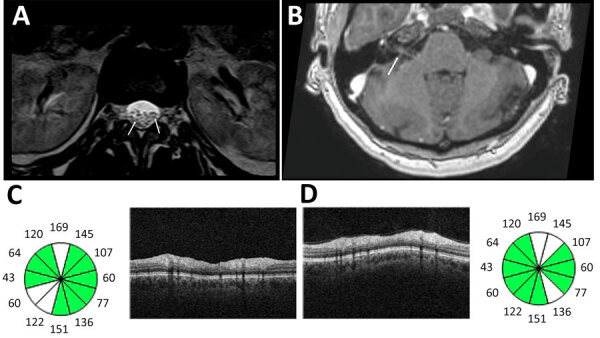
Imaging from study of Guillain-Barré syndrome and visual impairment associated with emerging Oropouche virus lineage, Brazil, 2024. A) T2-weighted magnetic resonance imaging of lumbar spinal cord. Arrows indicate nerve root edema. B) T1-weighted magnetic resonance imaging of brain with contrast. Arrow indicates enhancement of right facial nerve. C, D) Optical coherence tomography of right eye (C) and left eye (D) revealed bilateral loss of ganglionic cells in the papillomacular bundle, shown in white on the circle graphic.
